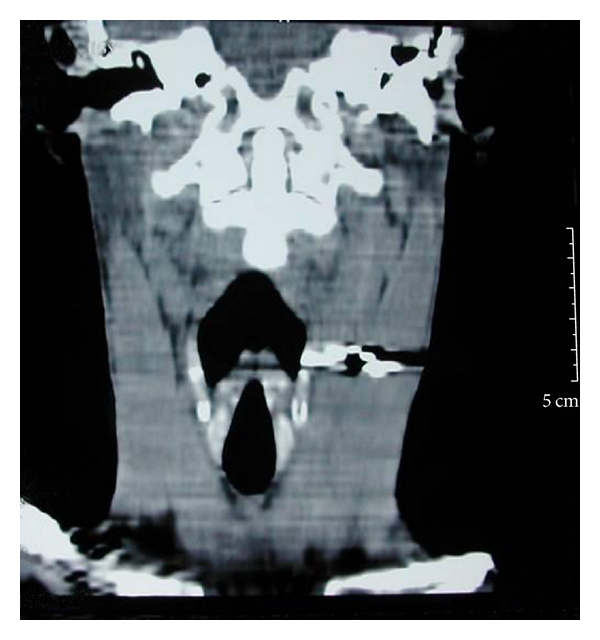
A coronal CT scan of neck showing the radio-opaque foreign body deep in the sternomastoid muscle.
